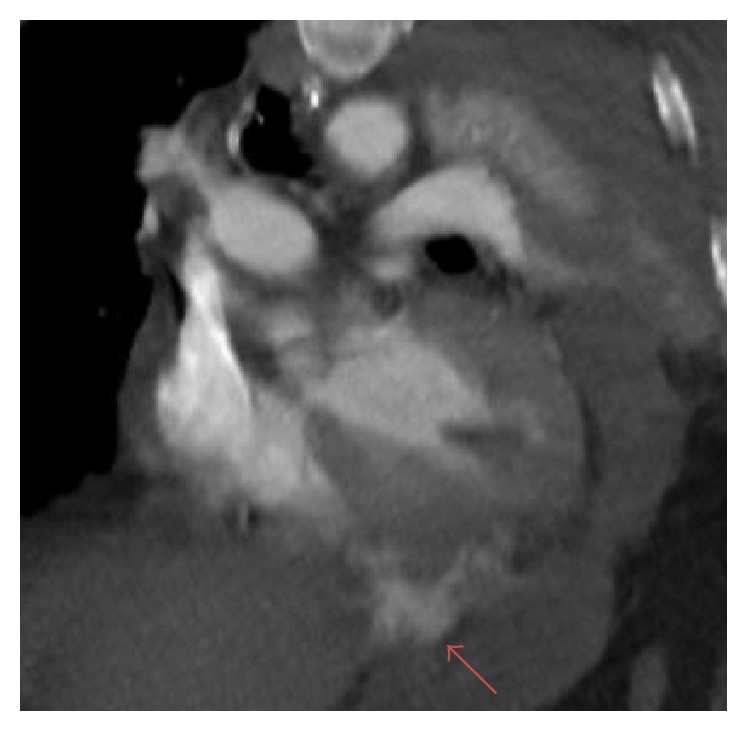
64-year-old male diagnosed with traumatic right ventricular rupture. Reformatted, coronal oblique contrast enhanced CT through the right and left ventricles demonstrates contrast extending from the right ventricle into the pericardium and intraperitoneal space (arrow).
